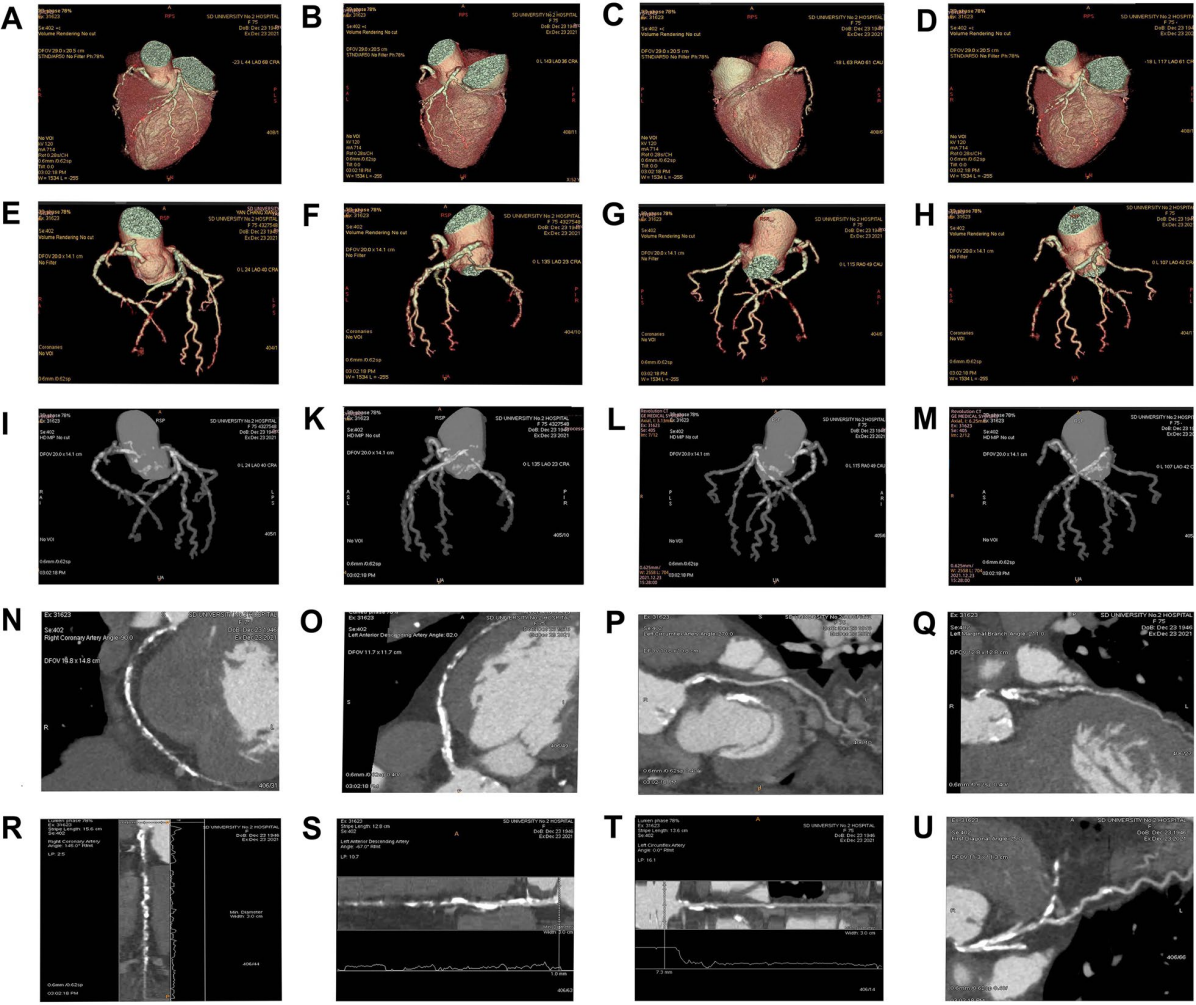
CTA images of a 75 year old woman with severe coronary calcification and disease. Different coronary CTA three-dimensional reconstruction techniques were used: A–D, VRT (volume rendering technique) for the whole myocardium and coronary arteries. E–H, VRT for coronary vascular trees. I–M, MIP (maximum intensity projections) for coronary vascular trees. N–U, CPR (curved planner reconstruction) for the degree of coronary stenosis and calcification in each large coronary branch: right coronary artery (N and R); left anterior descending branch (O and S); left circumflex branch (P and T); left marginal branch (Q); first diagonal branch (U)
